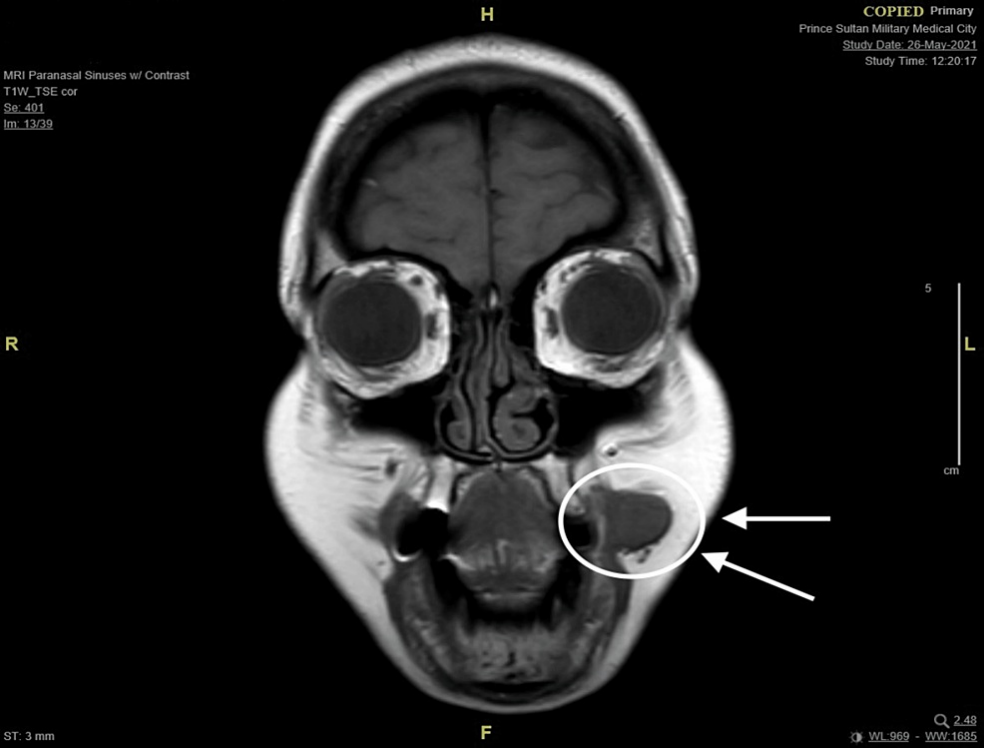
MRI T1-weighted image (coronal cut) demonstrating iso-intense mass on the left buccal space. MRI: magnetic resonance imaging
